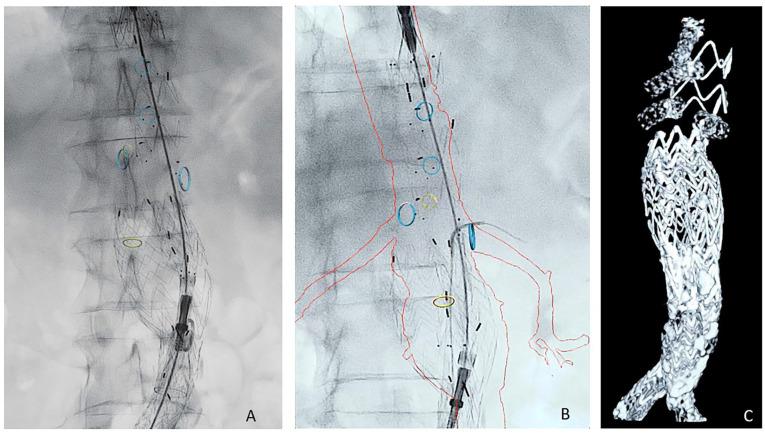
Treatment of a type 1a endoleak by placement of fenestrated TREO endograft in an earlier infrarenal abdominal endograft. (A) Partial deployment of the fenestrated TREO endograft, while focusing on the fenestrations (using the overlay of the vessel navigator), and anterior and posterior markers on the fenestrated TREO endograft. (B) Cannulation of the left renal artery. (C) SMART-CT at the end of the procedure showing the final result. CT, computed tomography.
